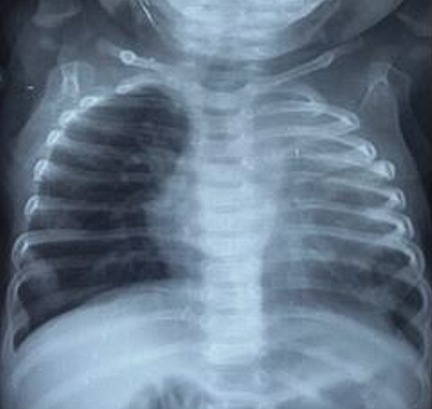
Radiographie du thorax postopératoire
